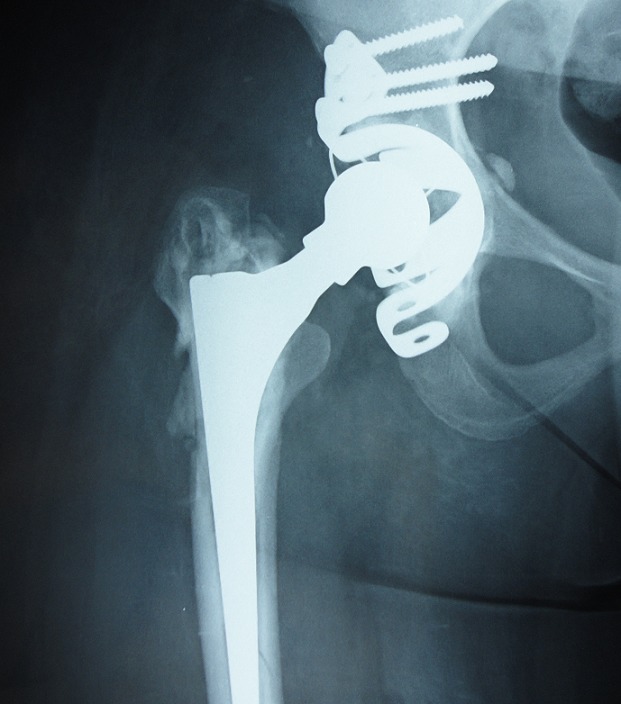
Radiographie de contrôle après reprise avec recèlement et reconstruction du cotyle par la mise en place de la croix de Kerboull
